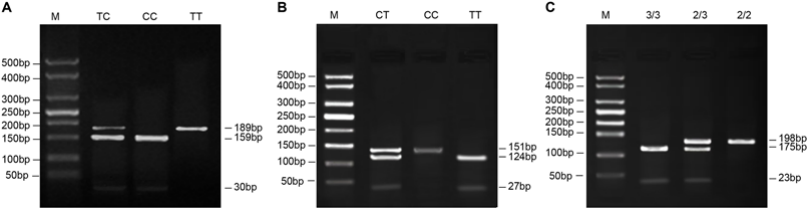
Electrophoresis maps of *EZH2* rs12670401, *EZH2* rs6464926, and *SMYD3*VNTR gene polymorphisms (A) Electrophoresis maps of *EZH2* rs12670401 gene polymorphisms. M, DNA marker; TC, TC heterozygotes digestion products, 189, 159, 30 bp; CC, CC homozygotes digestion products, 159 and 30 bp; TT, TT homozygotes digestion product, 189 bp. (B) Electrophoresis map of *EZH2* rs6464926 gene polymorphisms. M, DNA marker; CC, CC homozygotes digestion products, 151 bp; TT, TT homozygotes digestion products, 124 and 27 bp; CT, CT heterozygotes digestion products, 124, 151, and 27 bp. (C) Electrophoresis map of *SMYD3* VNTR gene polymorphisms. M, DNA marker; 3/3, 3/3 homozygote digestion products, 175 and 23 bp; 2/3, 2/3 heterozygotes digestion products, 198, 175, and 23 bp; 2/2, 2/2 homozygotes digestion products, 198 bp.
